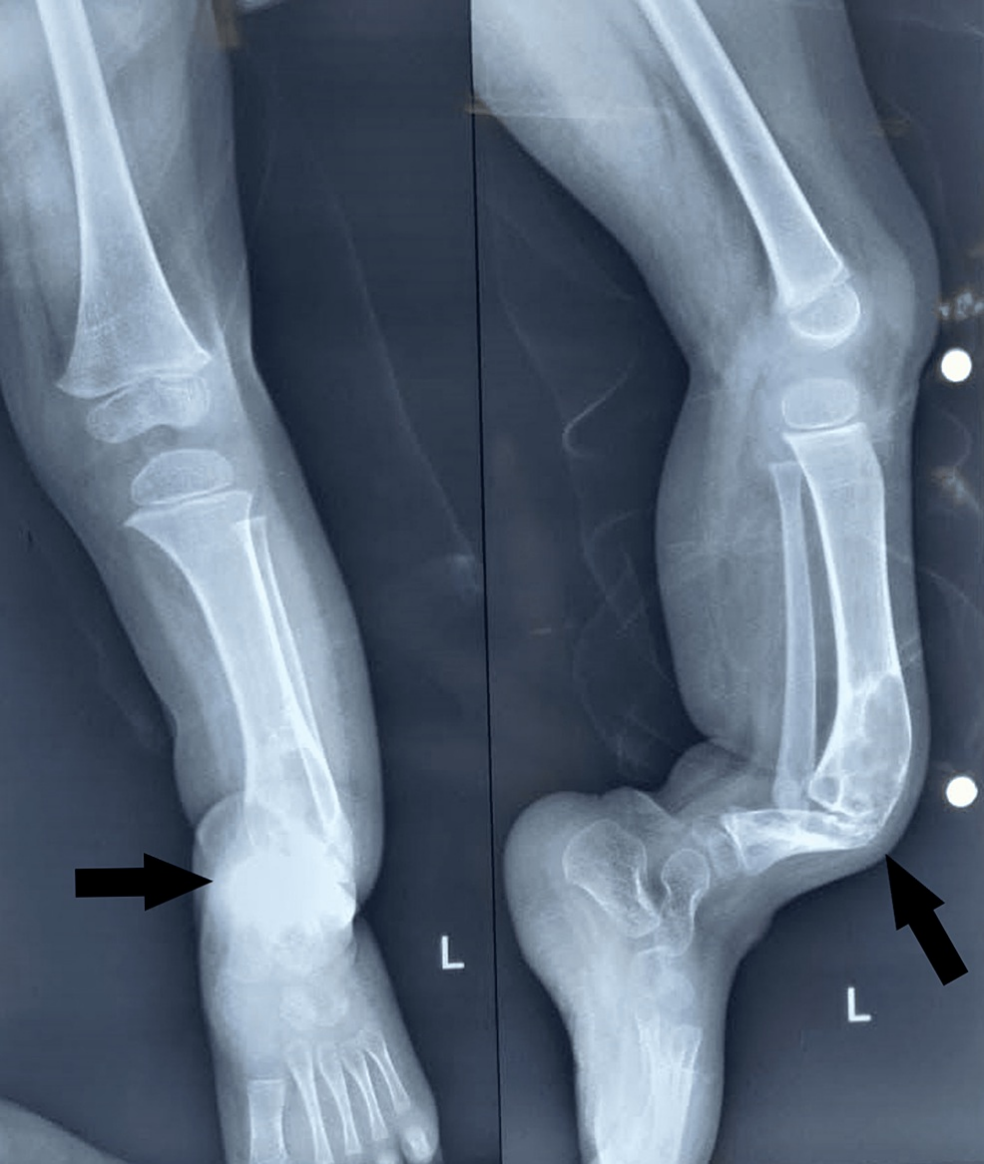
X-ray of the left tibia anteriorly (L) and laterally (R) showing pseudoarthrosis with signs of sclerosis The black arrows show the site of lesion on the tibia over the X-rays
